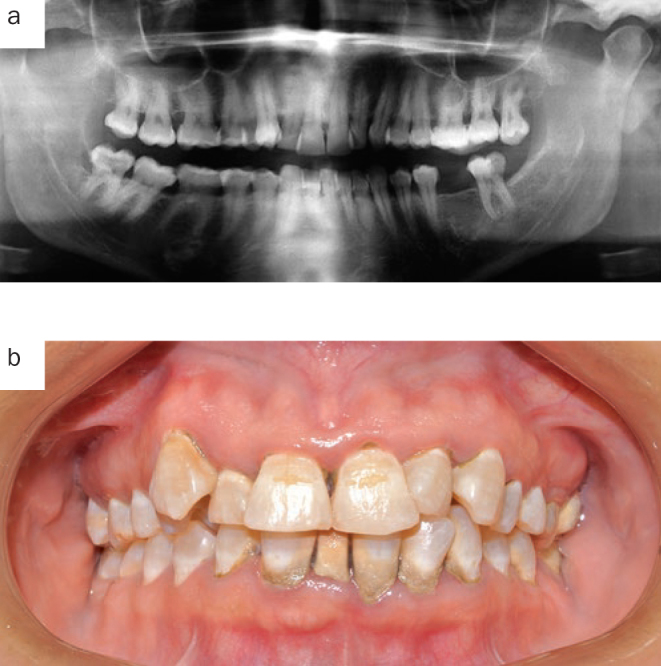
Patient 1, panoramic dental radiograph (a) and initial intraoral condition (b).
